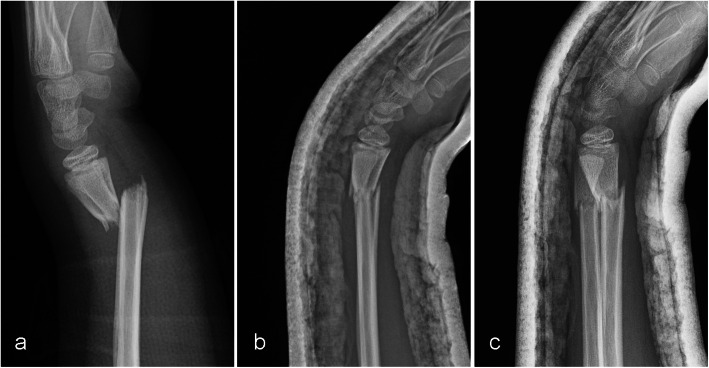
Lateral radiographs a before reduction, b after reduction, and c at the 1-week follow-up showing fracture redisplacement in an overweight child
